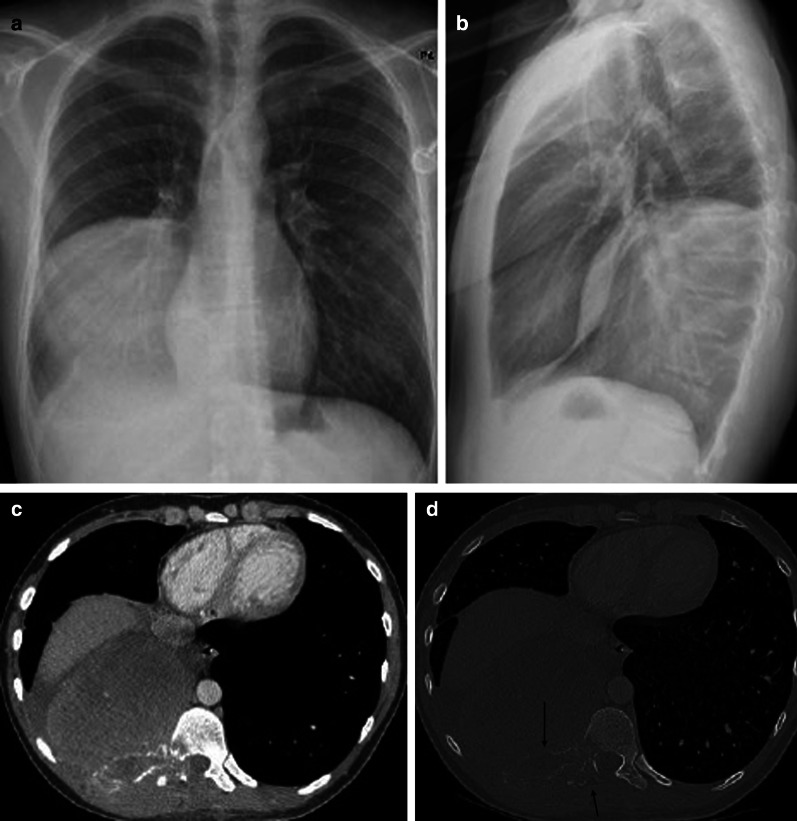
Diagnosis: extraskeletal Ewing sarcoma. Technique: standard chest radiography and contrast-enhanced chest CT. Description: A 17-year-old man presented with localized right-sided chest pain. Postero-anterior and lateral chest radiographs (a, b) show a well-delineated mass located posterior in the right lung. Axial contrast-enhanced CT (c) in the mediastinal window setting shows a heterogeneous mass with intralesional necrosis and focal bony destruction of the corpus of the vertebra, pedicle, and the posterior side of the rib (arrows), best seen in the bone window (d). The young age of the patient, locoregional bone destruction, and extensive soft tissue component is suggestive of an extraskeletal Ewing sarcoma
